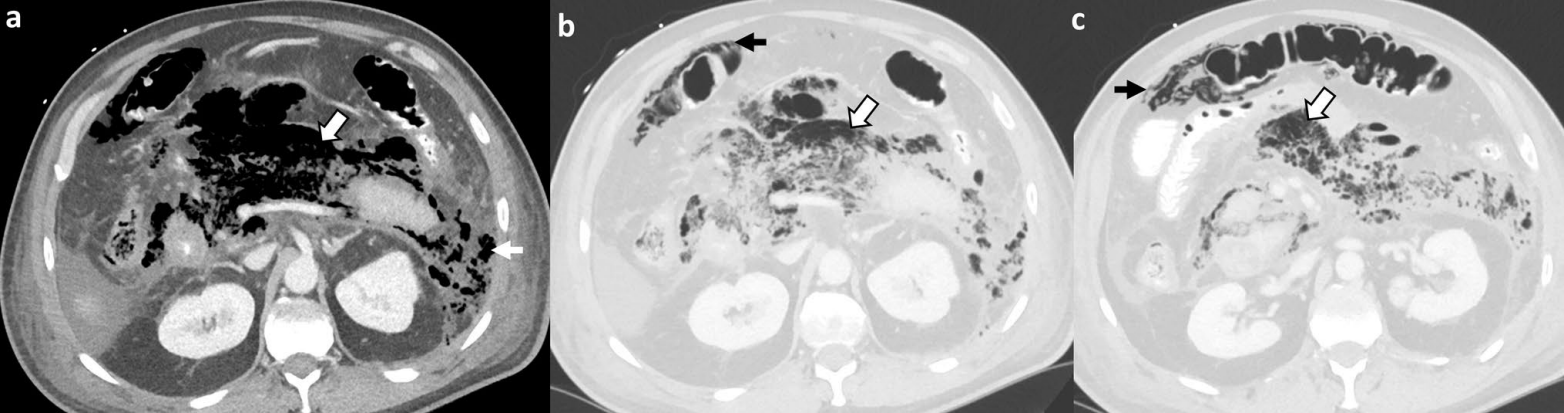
Emphysematous pancreatitis. A 64-year-old diabetic with alcohol abuse, with abdominal pain, elevated WBC counts, and elevated serum lactate levels. CT images show acute necrotizing pancreatitis with emphysema replacing the majority of the pancreas (white block arrows, a–c). Emphysema extends from the pancreatic bed/anterior pararenal space towards the left side (white arrow, a). There was also incidental benign pneumatosis coli in the transverse colon (black arrows, b, c), which resolved on follow-up CT
